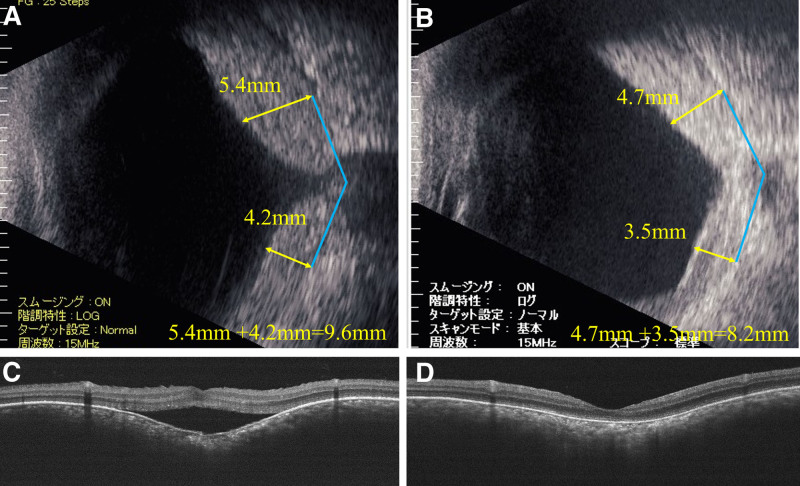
(A) B-mode ophthalmic ultrasound before proton beam therapy in the right eye. The height of the hemangioma was 9.6 mm (5.4 + 4.2 mm). (B) B-mode ophthalmic ultrasound 45 mo after proton beam therapy in the right eye. The height of the hemangioma was 5.7 mm (3.4 + 2.3 mm). (C) OCT before proton beam therapy. Mild retinal detachment was observed only in the macula. (D) OCT 45 mo after proton beam therapy. Retinal detachment improved. OCT = optical coherence tomography.
